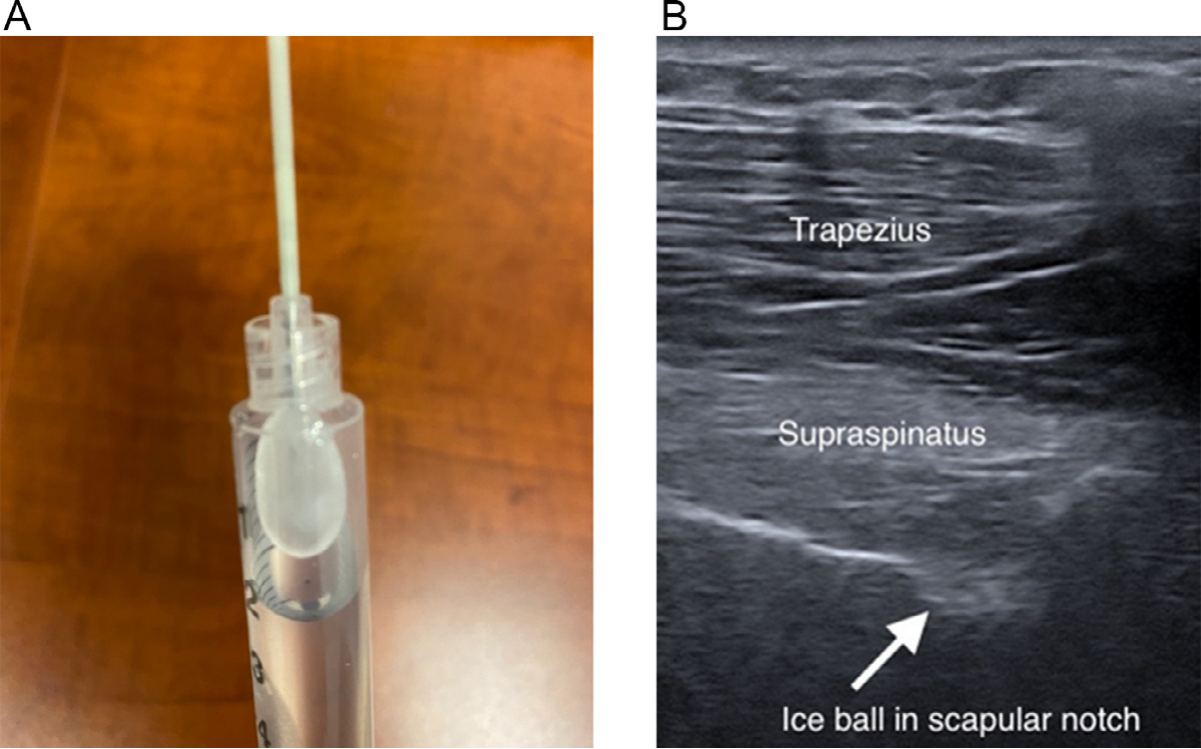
Equipment and perioperative ultrasonographic images of cryoneurolysis. (A) iovera system 190 Smart Tip, iovera, Pacira, NJ, USA, with ice ball created from water inside a syringe. (B) Fully formed ice ball during cryoneurolysis, visualized in the suprascapular notch with ultrasound.
